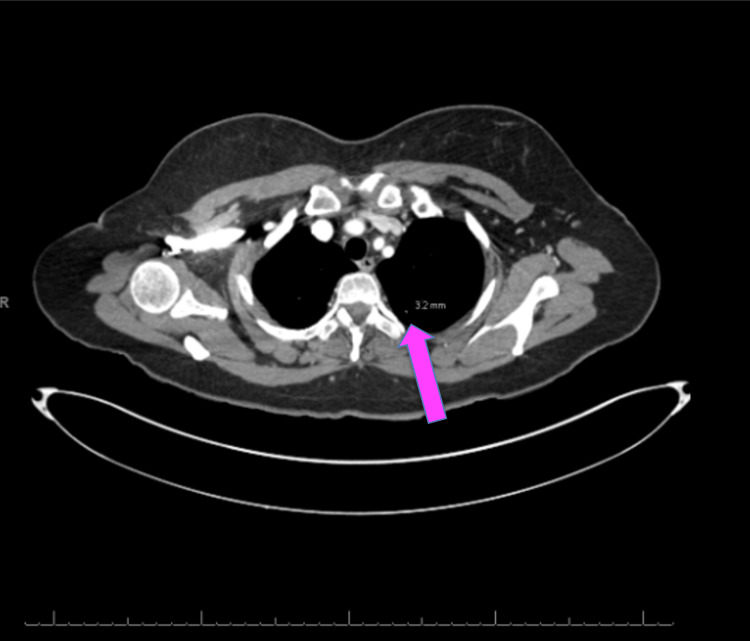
CT scan of the chest revealed left side pulmonary nodule
